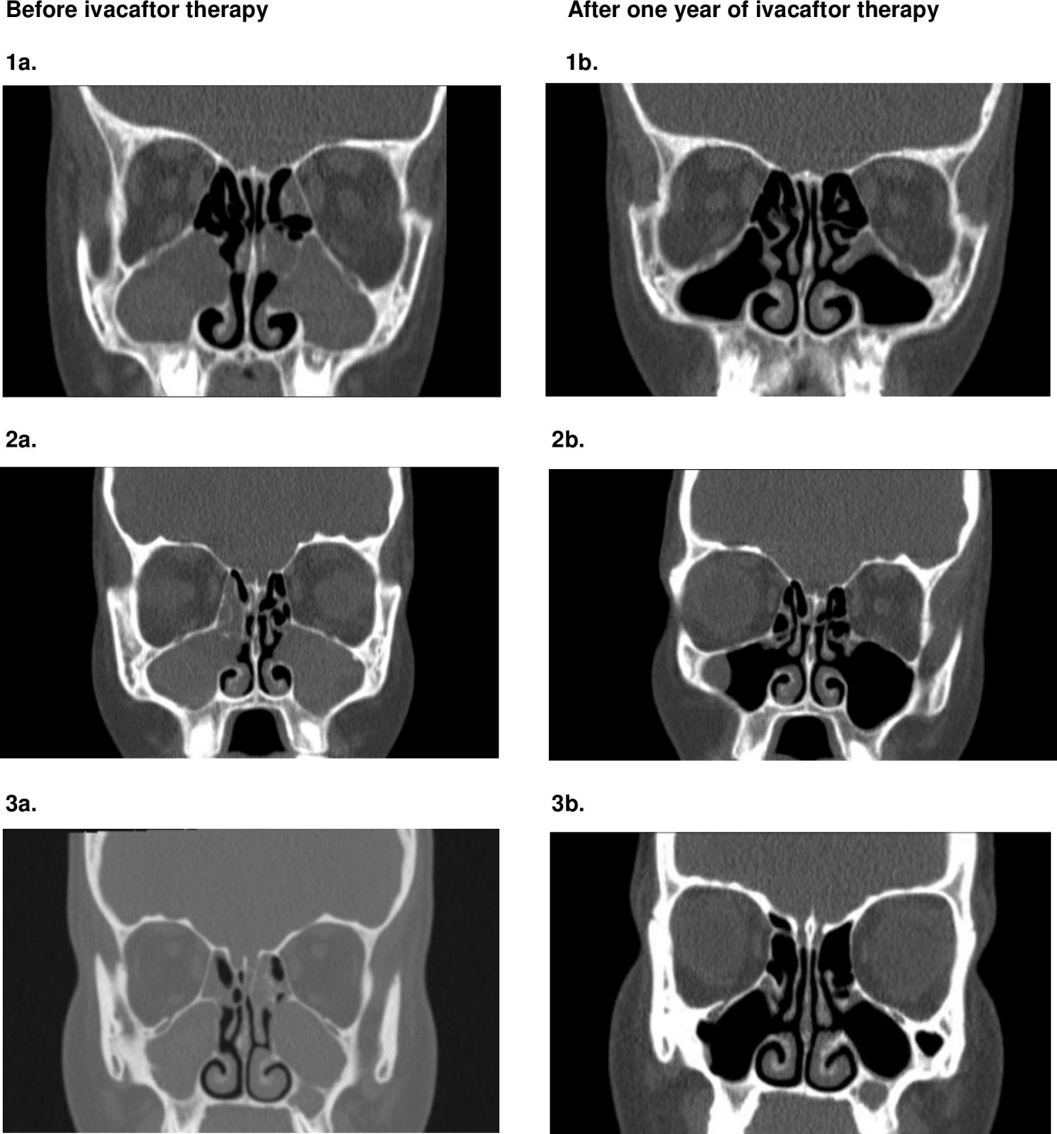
A couple of CT scans before and after one year of ivacaftor therapy.
